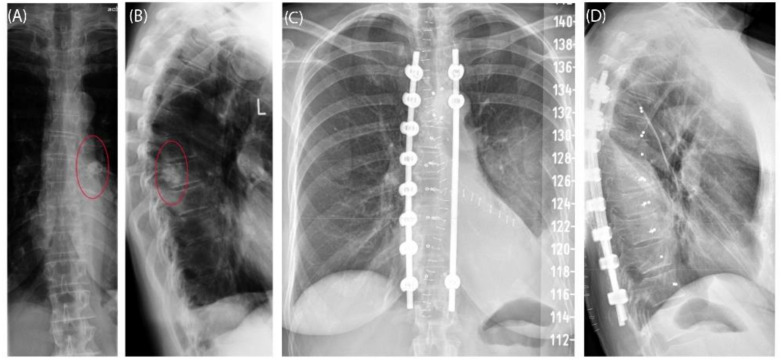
Case 3: Preoperative radiographs in frontal (A) and lateral (B) views, red circles indicate the bony tumor mass; postoperative radiographs in frontal (C) and lateral (D) views.
